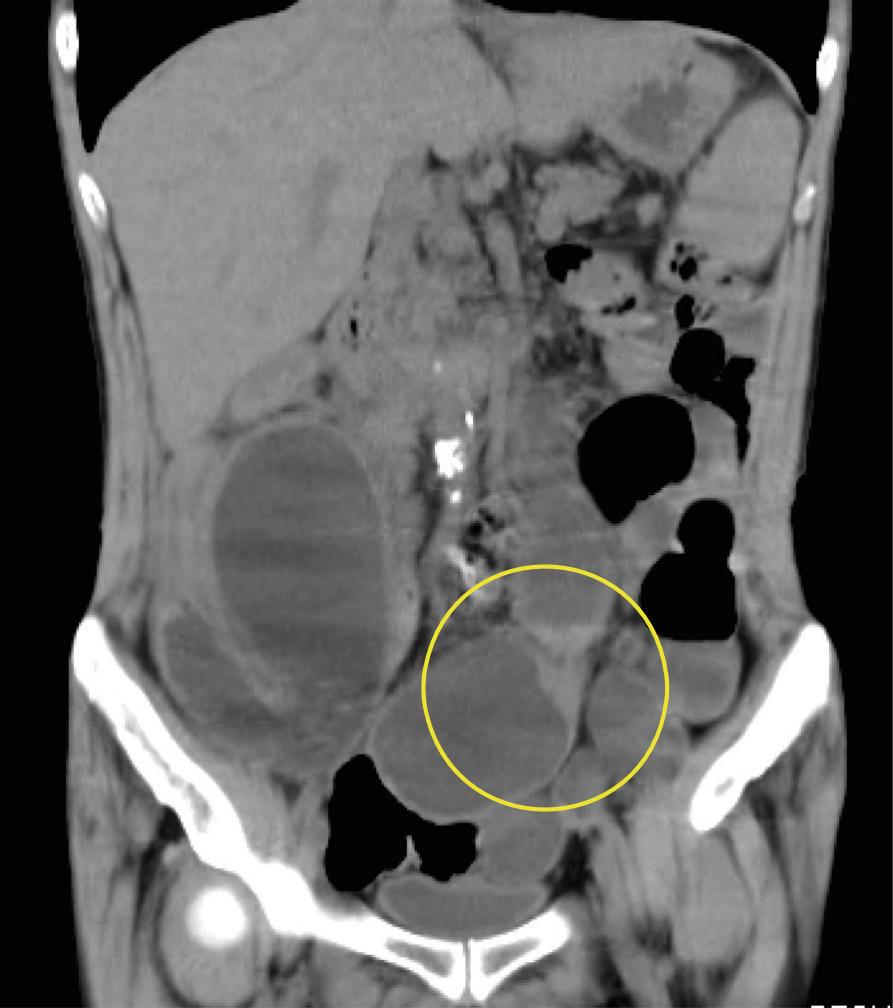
The wall of the small bowel is thick and stenosed, as seen on computed tomography (yellow circle)
